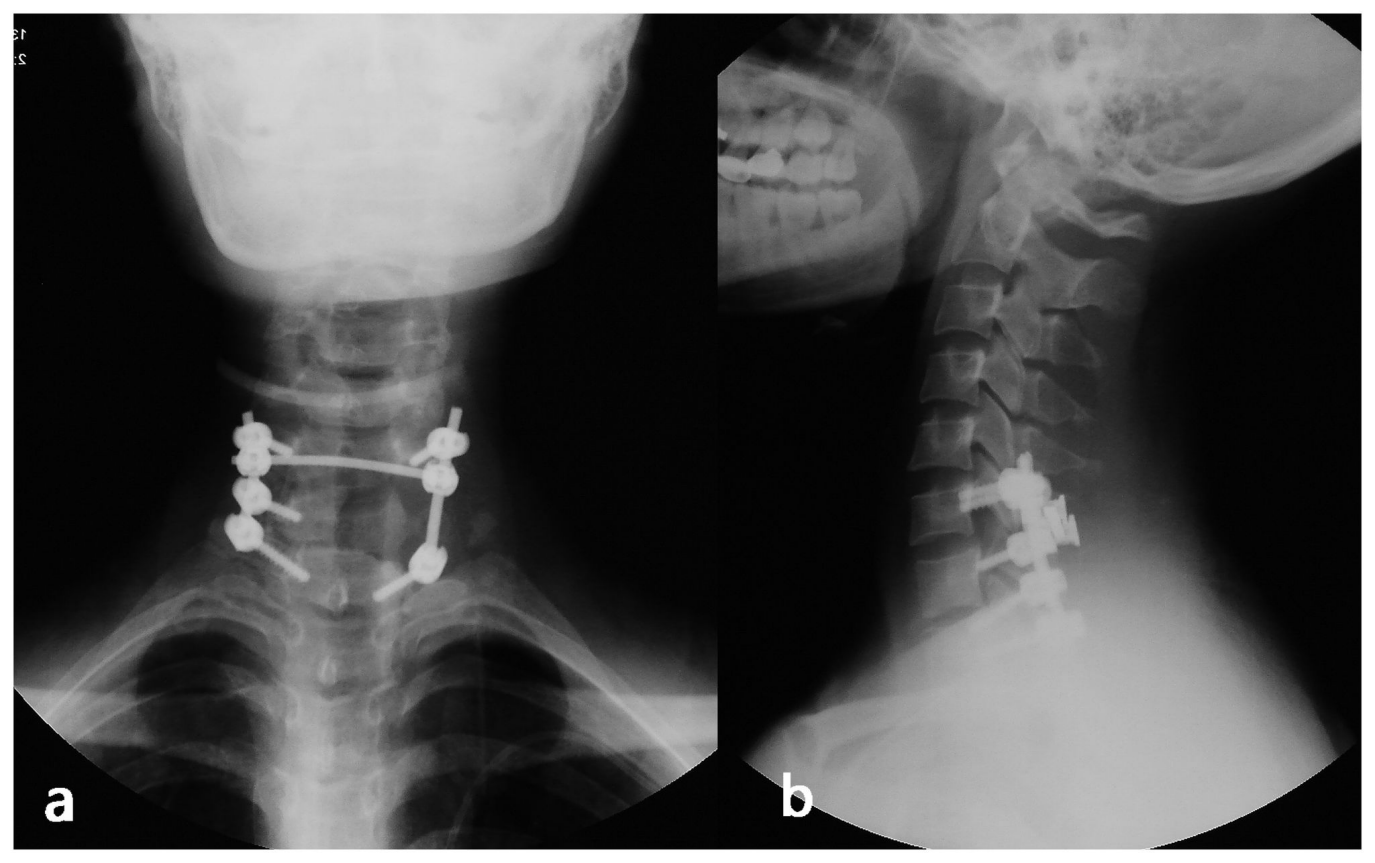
Postoperative AP and lateral radiographs of cervical vertebrae. a: anteroposterior radiograph; b: lateral radiograph. The patient underwent posterior cervical en bloc resection of the tumor, spinal cord decompression, pedicle screw internal fixation as well as spinal fusion using a bone graft.
